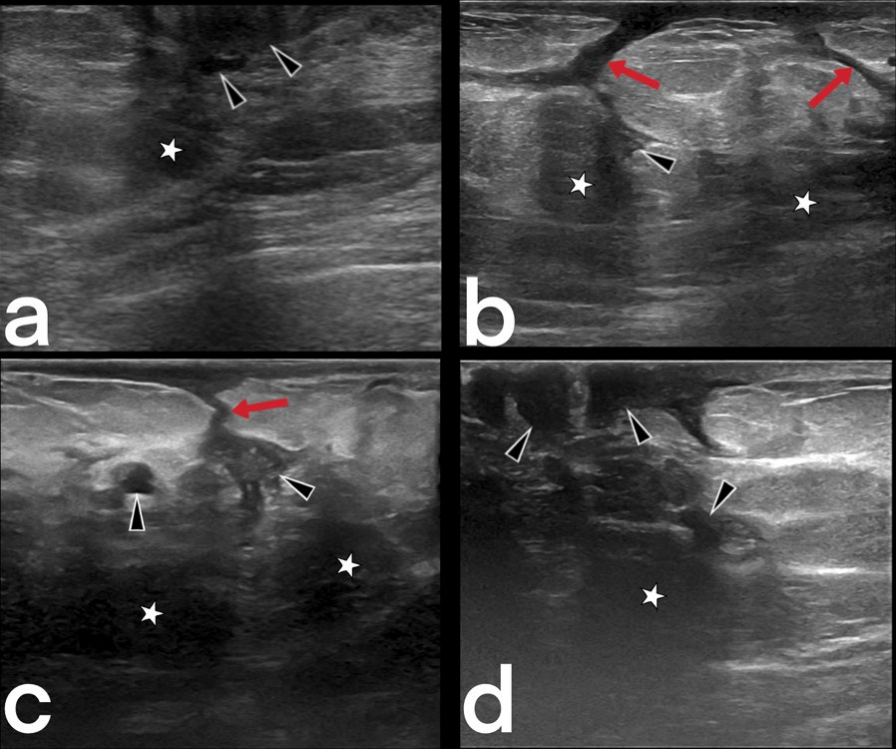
Ultrasound images from four different female patients (a–d). Abscesses (arrowheads), sinus tracts (arrows), and hypoechoic parenchymal edema areas (stars)
